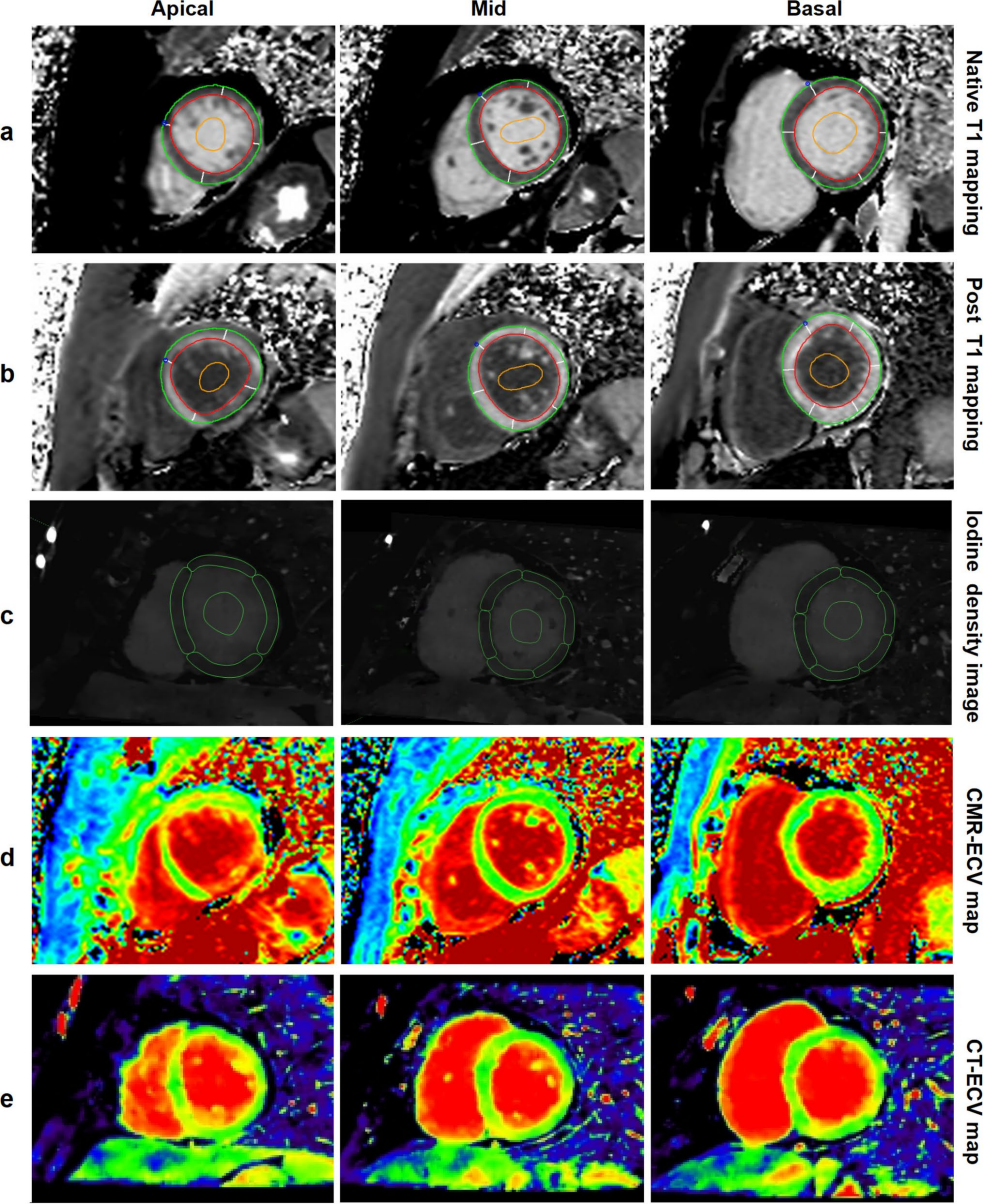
Regions of interest drawn on T1 mapping and iodine density images. a–c Regions of interest were manually drawn on left ventricular (LV) myocardium images from a control subject according to the American Heart Association’s 16-segment model and on the LV blood pool to calculate the extracellular volume fraction (ECV). Red outline = endocardium, green outline = epicardium, orange outline = blood pool. a Native T1 mapping: Native T1 values at the basal, mid, and apical layers of LV myocardium were 1072 ms, 1059 ms, and 1055 ms, respectively. b Post-contrast T1 mapping: Post-contrast T1 values at the basal, mid, and apical layers of LV myocardium were 427 ms, 406 ms, and 407 ms, respectively. c Iodine density images: Iodine density values at the basal, mid, and apical layers of LV myocardium were 0.88 mg/ml, 0.82 mg/ml, and 0.81 mg/ml, respectively. d CMR-ECV mapping: CMR-ECV at the basal, mid, and apical layers were 25.19%, 25.27%, and 25.88%, respectively. e CT-ECV mapping: CT-ECV at the basal, mid, and apical layers were 26.01%, 24.12%, and 24.18%, respectively. The global CMR-ECV and CT-ECV values were 25.45% and 24.77%, respectively
